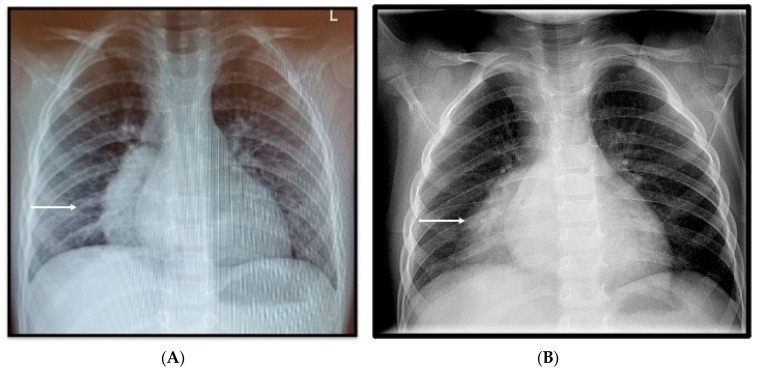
Frontal chest X-ray (A). Initial outpatient X-ray performed at another medical facility, a day prior to admission, reveals large dense paracardial opacity (white arrow); (B). Day 1 of hospital admission, showing inhomogeneous right para- and retrocardiac ill-defined dense opacity and air bronchogram (white arrow).
